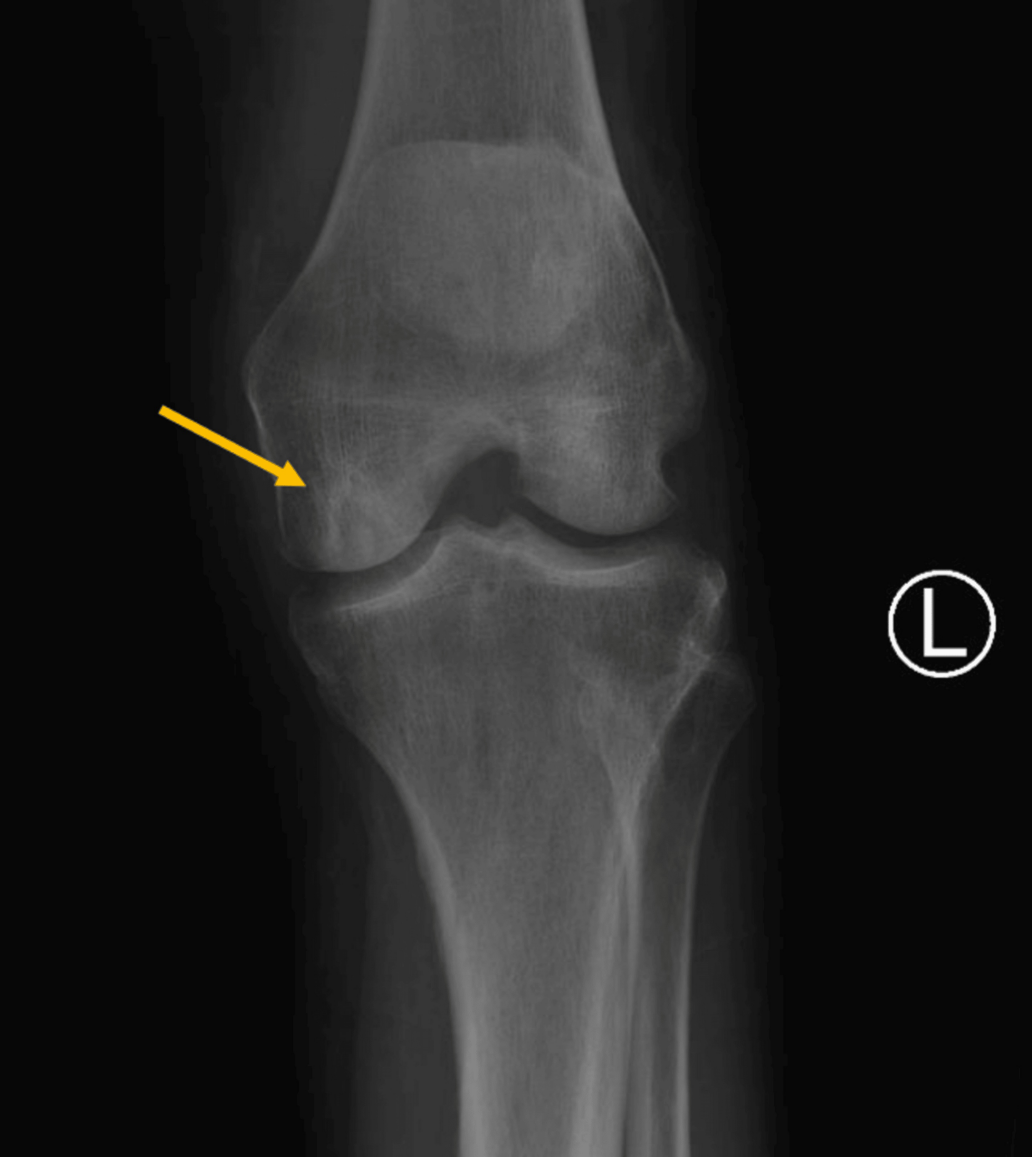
Left knee X-ray depicting a sclerotic lesion in the medial femoral condyle (yellow arrow).
